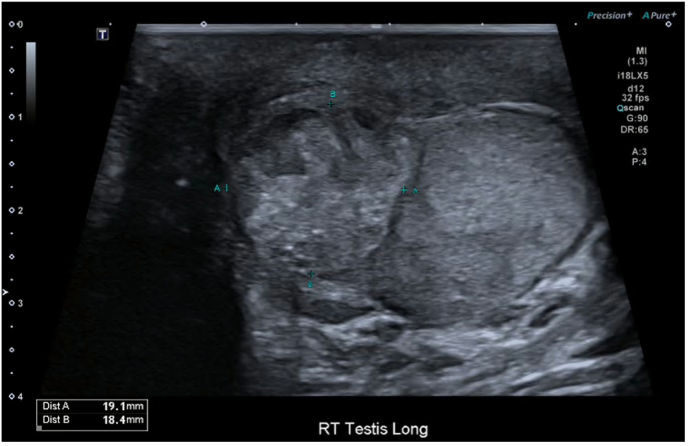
Longitudinal ultrasound of right testicle.
